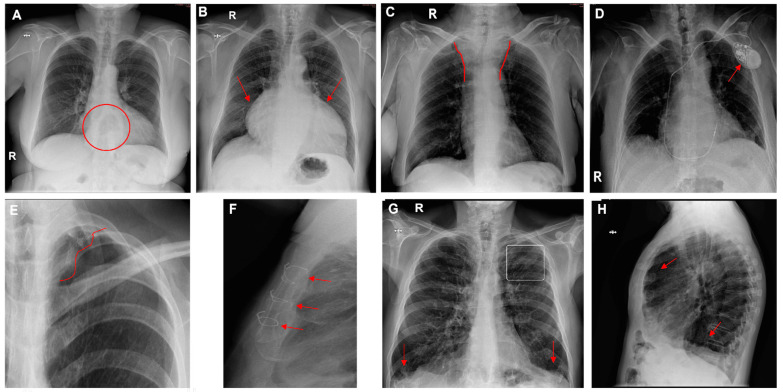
Chest radiographs with additional findings highlighted by red circles, arrows, or lines. (A) Hiatal hernia, (B) cardiomegaly, (C) widening of the superior mediastinum, (D) heart pacemaker, (E) pulmonary surgical material, (F) sternal surgical material, (G,H) pulmonary hyperinflation (G—flattening of the diaphragms and enlarged retrosternal space; H—enlarged retrocardiac spaces).
